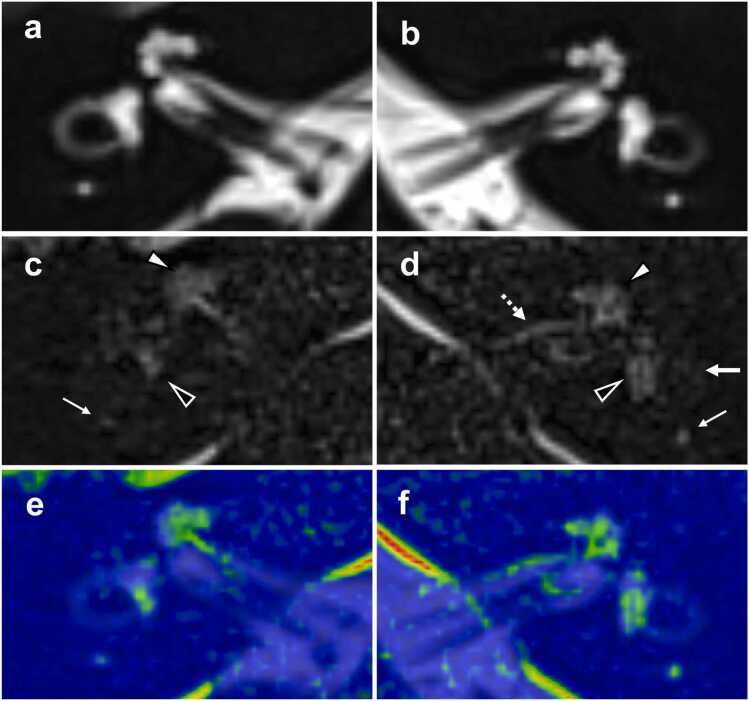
68-year-old male with inner ear high signal on non-contrast 3D FLAIR imaging, associated with bilateral tinnitus. a-b MRC: The inner ear lymph on the right (a) and left (b) sides demonstrates hyperintensity. c-d Non-contrast HT2-FLAIR imaging: Hyperintense signals are observed in the lymphatic fluid of both inner ears, including the cochlea (white arrowhead), vestibule (black arrowhead), posterior semicircular canal (small arrow), and lateral semicircular canal (arrow). Abnormal signals, all rated as grade 1, seem to be present within the perilymph. Notably, linear hyperintensity is evident within the left internal auditory canal (dotted arrow), indicative of dural/subdural lesions. e-f Fusion image generated from MRC and non-contrast HT2-FLAIR image. Abbreviations: 3D *three-dimensional*, FLAIR *fluid attenuated inversion recovery*, MRC *MR cisternography*, HT2-FLAIR *heavily T2‑weighted 3D FLAIR*.
